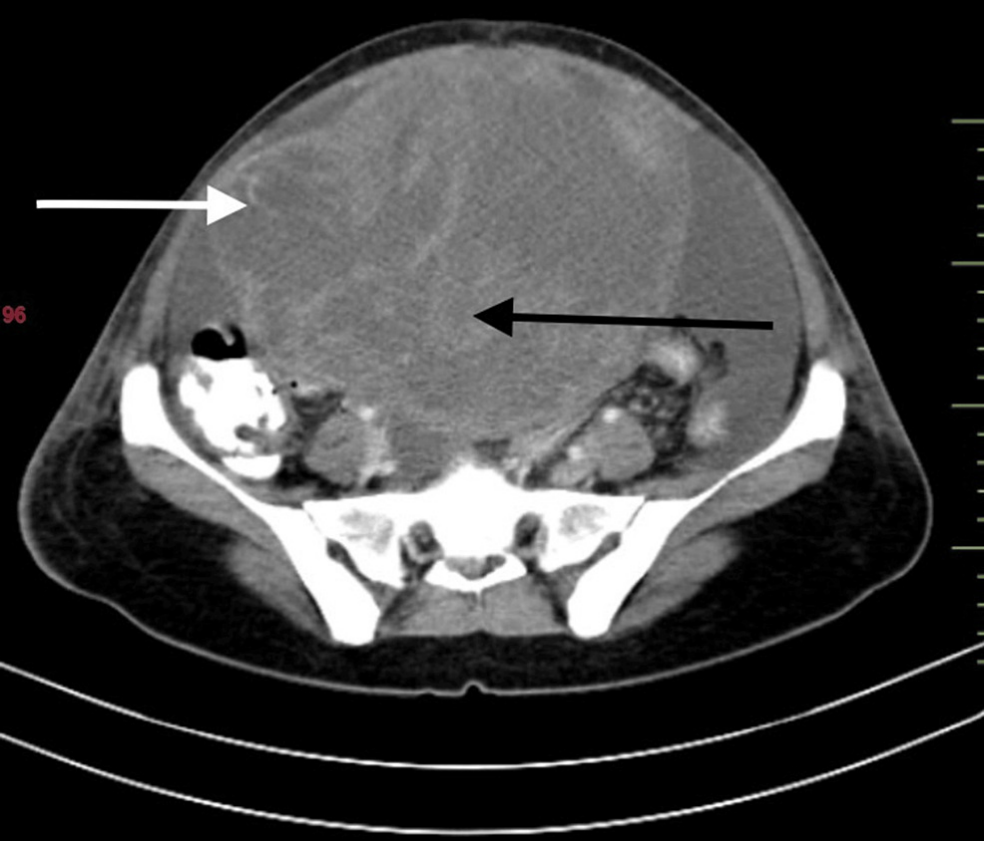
Contrast-enhanced CT abdomen and pelvis axial section showing a solid (black arrow)-cystic (white arrow) mass
